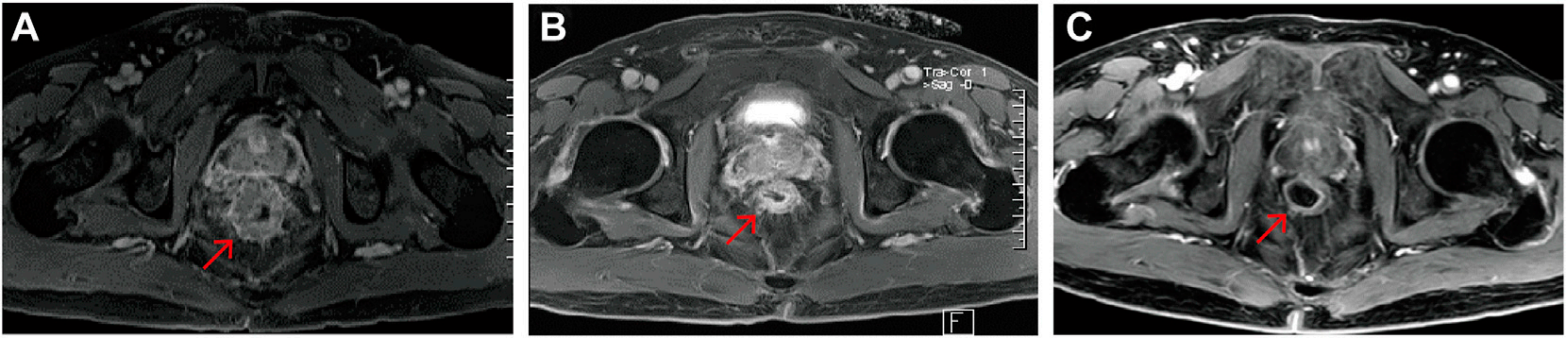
Rectal magnetic resonance images of the POLE-mutant rectal cancer patient receiving camrelizumab and anlotinib. (A) Pre-immunotherapy; (B) 7 months post—immunotherapy; (C) 18 months post—immunotherapy. Red arrows indicate the same rectal tumor lesion.
